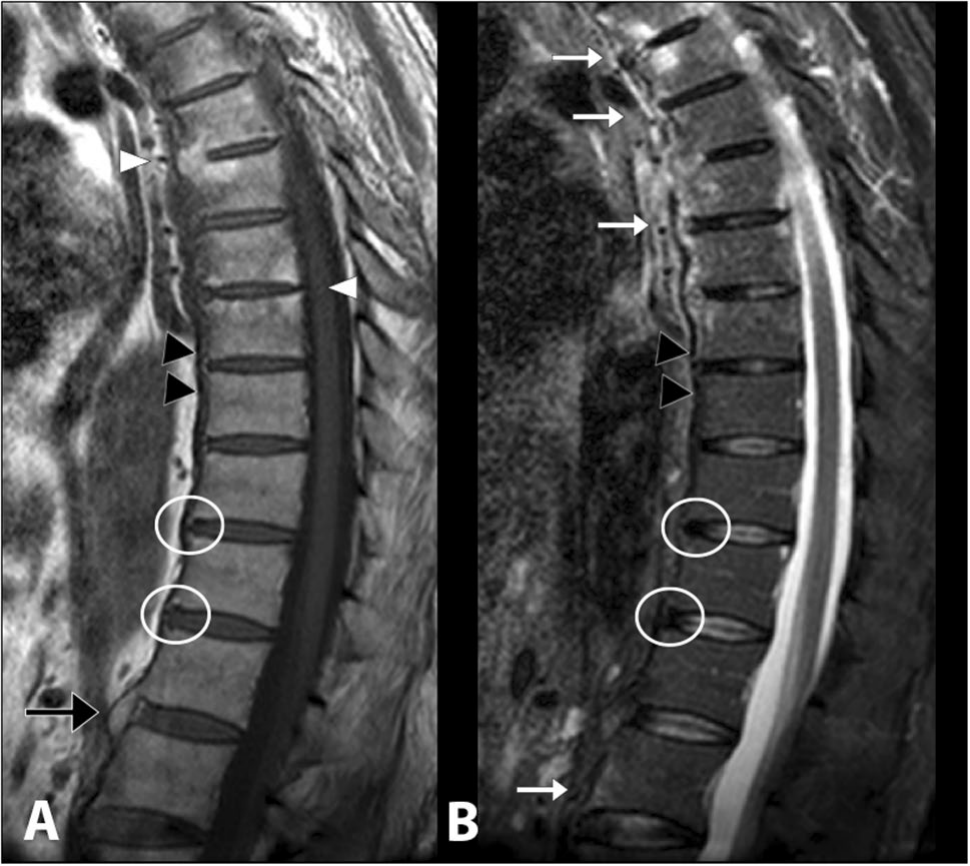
Sagittal T1-weighted image (a) and sagittal STIR image (b) showing hypointense and hyperintense lesions, respectively, at the anterior edges of vertebral bodies from D3 to D8 and in L1, indicating bone marrow edema (Romanus lesions) (white arrows). The post-inflammatory areas of fatty degeneration of the bone marrow are visible at the anterior edges of the opposite vertebral bodies D5/D6 and at the posterior edges of D7 and D8 (white arrowheads). Thickening of the anterior longitudinal ligament (black arrowheads) and multiple syndesmophytes (white circles) can also be detected. Furthermore, in D12-L1 it is possible to observe a bony bridge (black arrow), which could result from the progression of ossification on syndesmophytes
